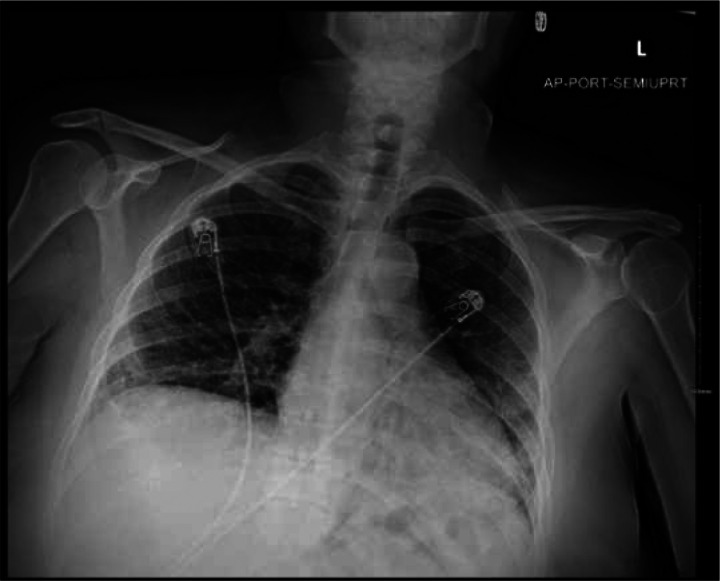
Portable chest X-ray on presentation to the Emergency Department (admission 1, day 1).
